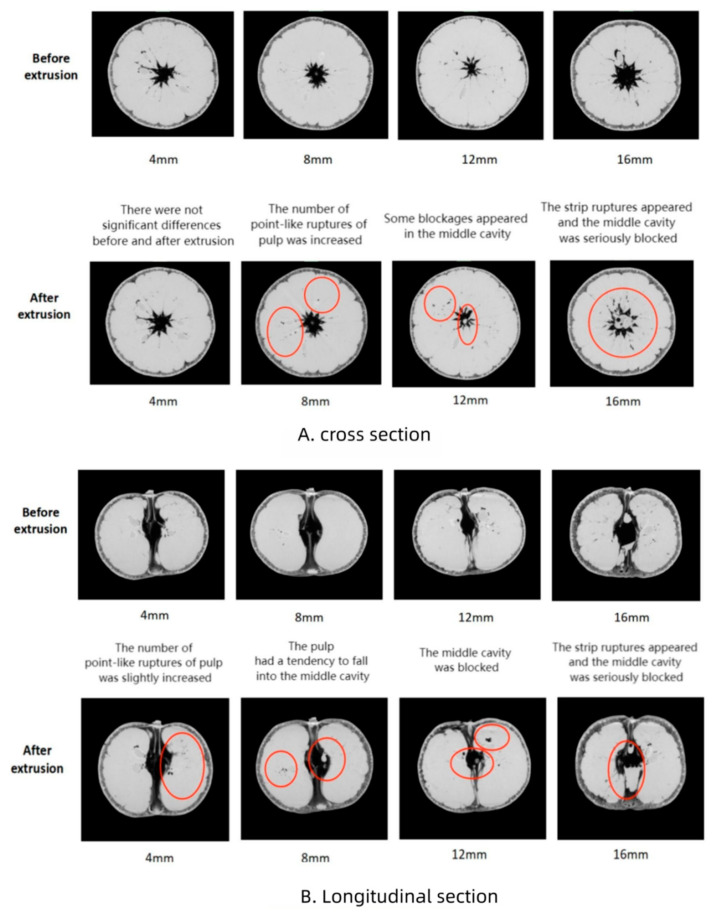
CT scan images of mandarins. The black font in the middle of the picture shows the change of the citrus pulp before and after compression, and the red circle is a mark for highlighting.
